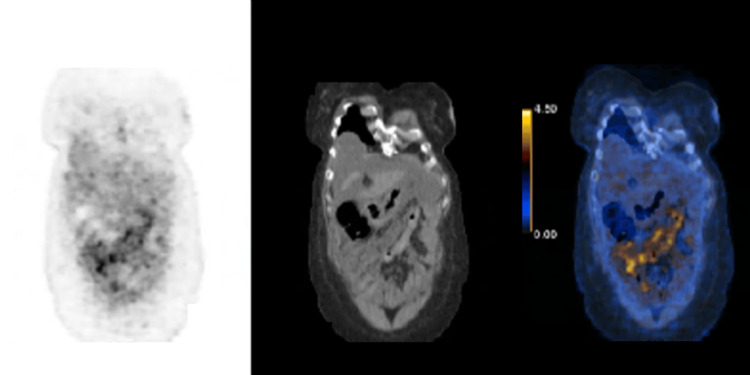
18F-fluorodeoxyglucose (FDG) positron emission tomography scan revealed extensive diffuse hypermetabolic peritoneal densifications.
